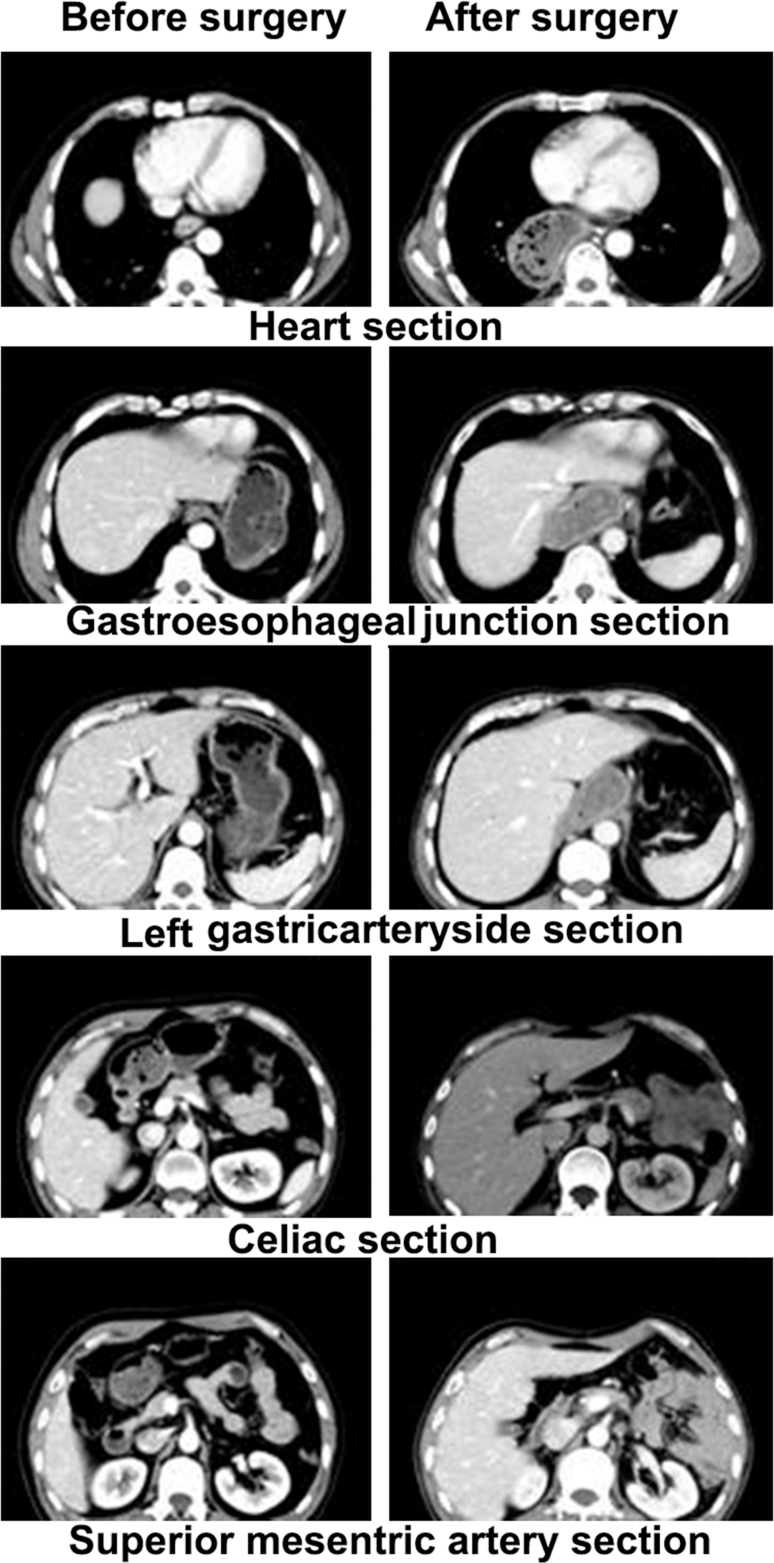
CT images of the inferior mediastinum and epigastrium before and after the radical surgery.
